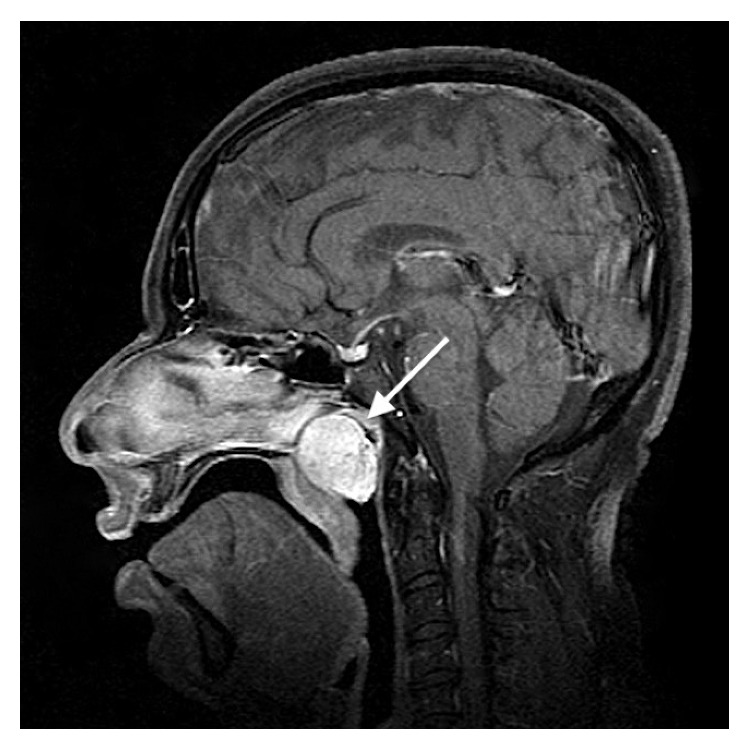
Sagittal T1-weighted MR image showing a well-defined enhancing tumour (arrow) completely filling the nasopharynx.
